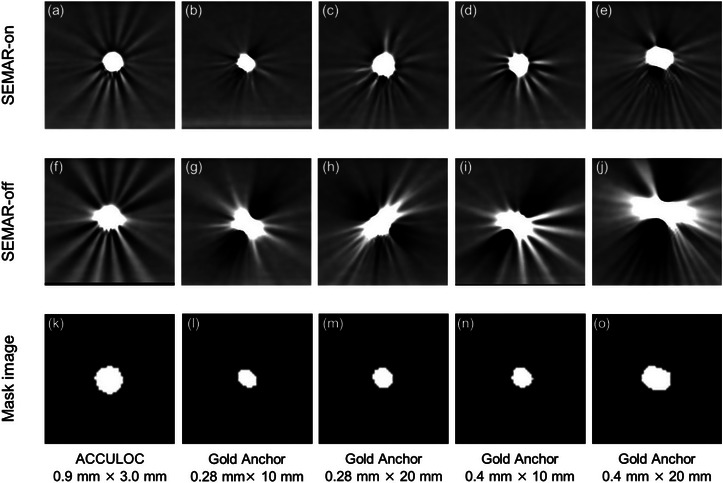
Representative axial slice of CT images for each fiducial marker. (a–e) represent CT images reconstructed with SEMAR‐on algorithm for each fiducial marker. (f–j) represent CT images reconstructed with SEMAR‐ off algorithm for each fiducial marker. (k–o) represent mask image of each marker. The masked region has intensity values greater than 250 HU in CT images.
